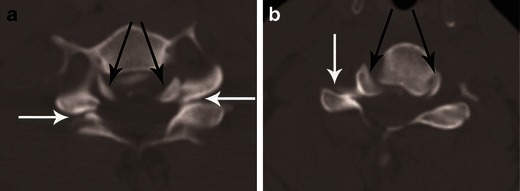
Bilateral versus unilateral facet joint dislocation. a Axial CT of the C5–C6 facet joint shows bilateral facet joint dislocation, suggested by reversal of the normal facet relationship, with convex surfaces opposing each other—called the “reverse hamburger sign” (*white arrows*). Loss of concentric relationship of the uncinate processes (*black arrows*) to the superior vertebral body—called the “positive headphone sign”. b Axial CT of the C4–C5 facet joint shows unilateral right-sided facet joint dislocation, suggested by a “naked facet” (*white arrow*)—due to absence of the opposing facet joint articular process because of dislocation. Loss of concentric arrangement of the right uncinate process (*black arrows*) due to rotation of vertebral body to the undislocated left side, resulting in unilateral positive “headphone sign”—suggesting rotational deformity
